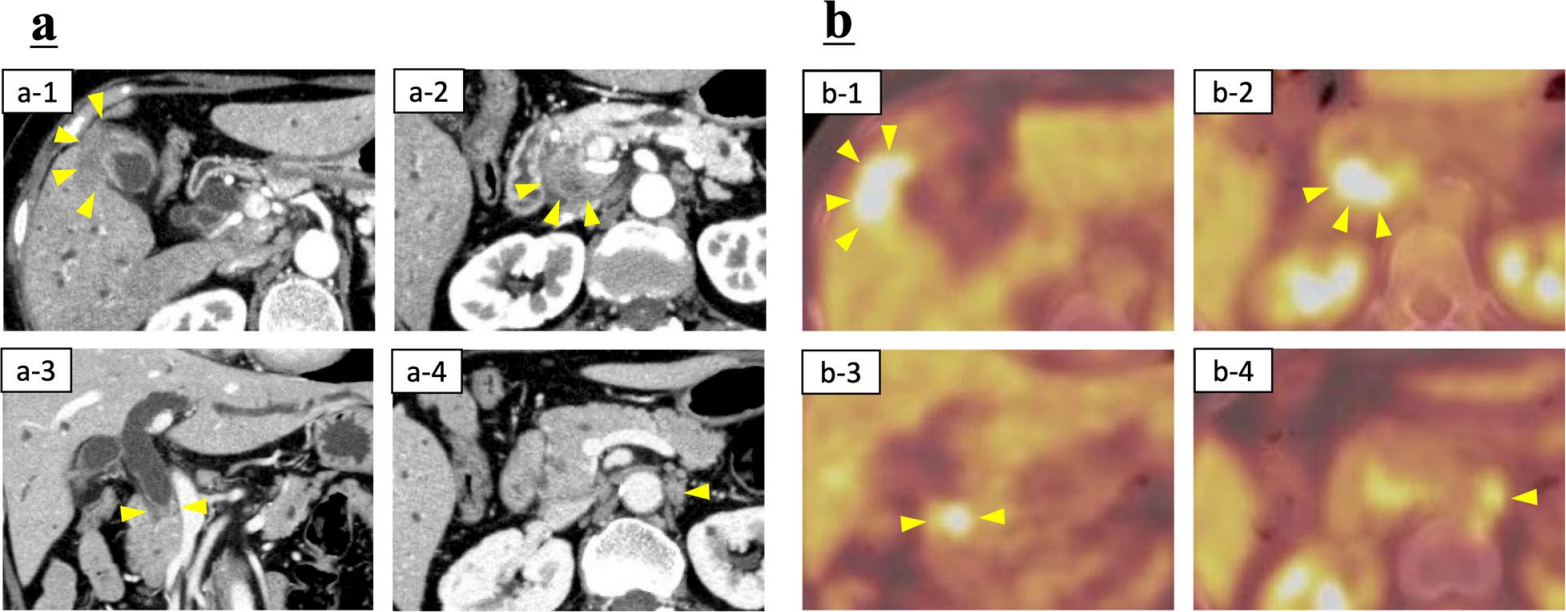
a Multidetector-row computed tomography findings, and the lower row shows the corresponding positron emission tomography. a-1 The tumor at the fundus of the gallbladder infiltrating the liver parenchyma. a-2 Bulky metastasis of the hepatoduodenal mesenteric lymph node. a-3 Bile duct stenosis due to bile duct infiltration of the metastasis lymph node, along with dilation of the intrahepatic and extrahepatic bile ducts. a-4 Metastasis of the para-aortic lymph node. b Positron emission tomography findings corresponding to a
